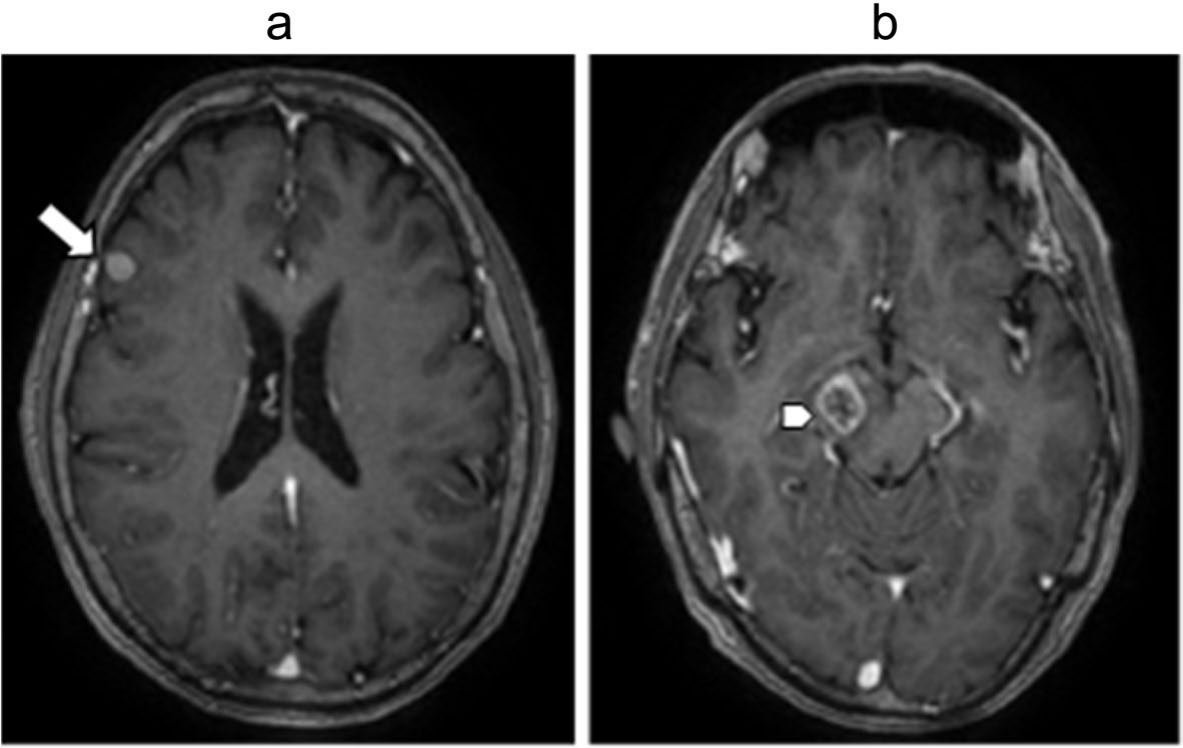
Brain metastases lesions in MRI T1-weighted after contrast enhancement (a): The right frontal cortex lesion (arrow). (b): The right cerebral peduncule lesion (arrowhead)
